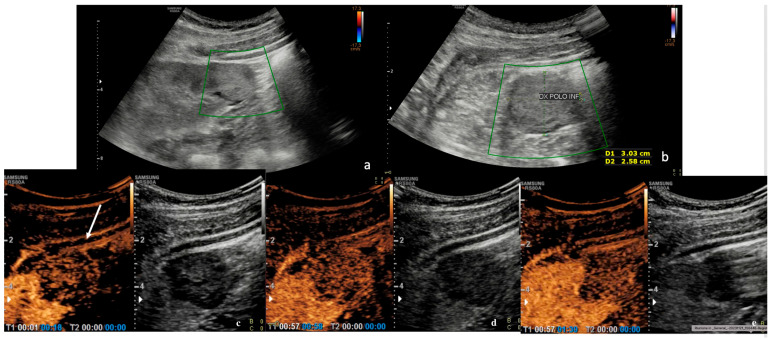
(a,b) B-mode ultrasound shows a solid exophytic hyperechoic renal lesion at the right lower renal pole. CEUS. During the arterial phase (c) (18 s), the lesion appeared hypovascular compared to adjacent renal parenchyma. (d,e) In the venous and late phase (58 s to 1.30 min), the lesion showed rapid and inhomogeneous wash-out compared to the adjacent renal parenchyma (white arrow).
